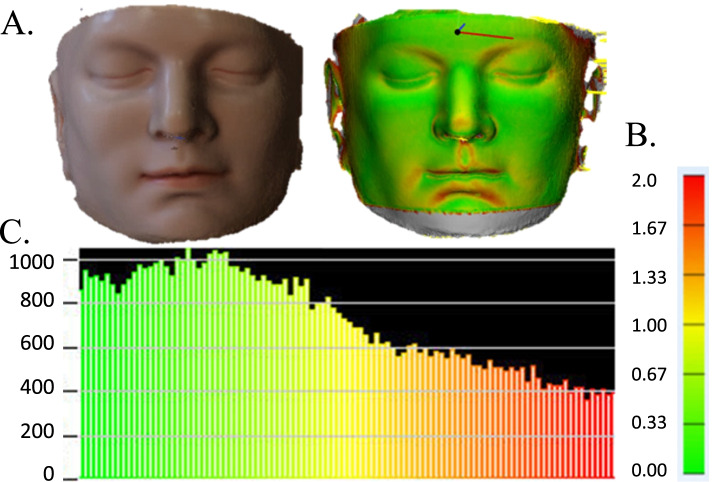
Part comparison analysis of measurements determined by structed light scanning camera (Bellus) compared to CT scanned surface map. a) The structured light scanning 3D data was superimposed onto the CT scanned image surface and the comparison to the 3D model was assessed by color mapping. b) Color scale representing the difference in mm between each point of the 3D surface with the corresponding CT surface data. Green = 0.0 mm difference. Red = 2.0 mm difference. c) A histogram depicting the number of points that correspond to each color
